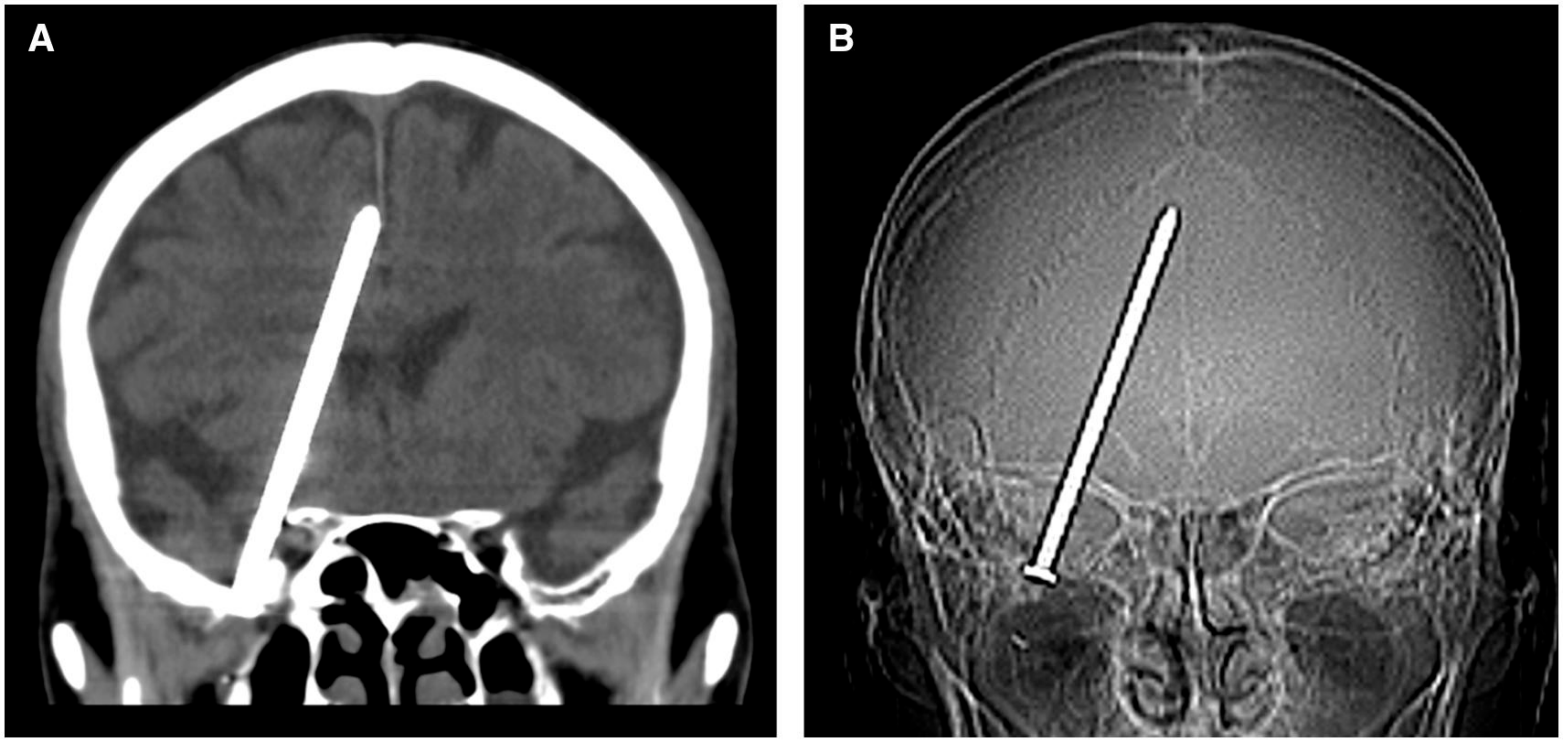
CT shows a nail inside the patient’s cranial cavity. (A) Coronal CT image and (B) CT scout image.
